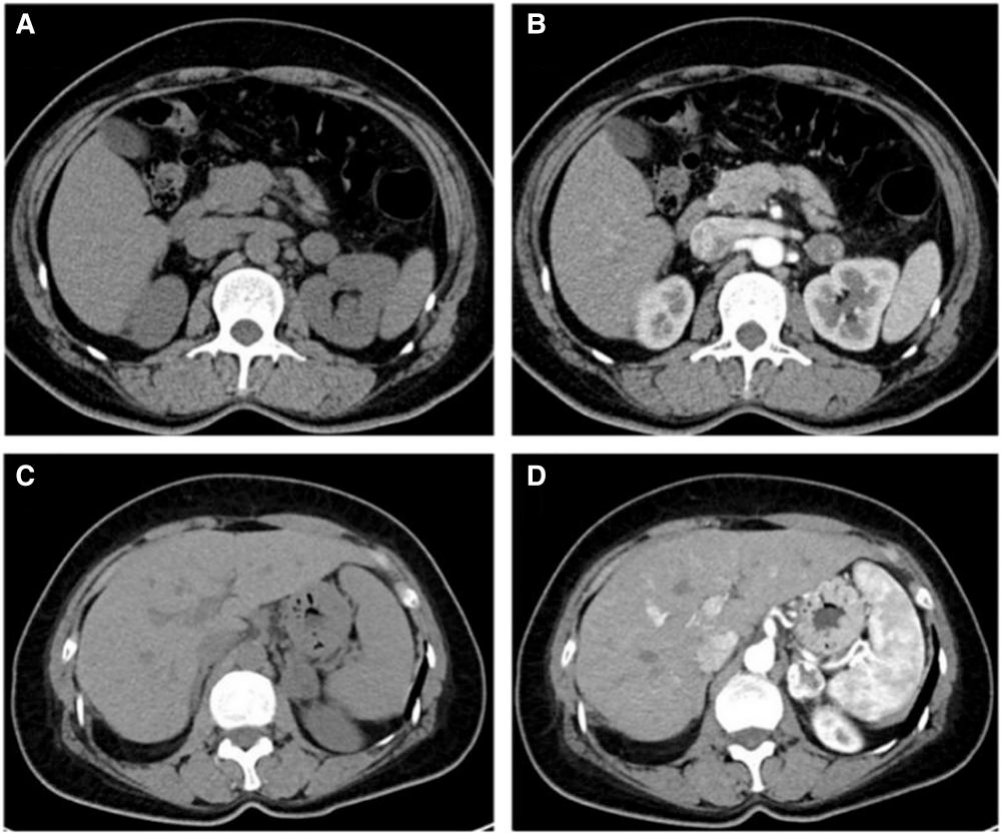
Contrast-enhanced computed tomography demonstrating different enhancement patterns of different size tumors. (A, B) shows mild enhancement of a 1.8 cm tumor and (C, D) shows marked enhancement of a 2.3 cm tumor in the arterial phase.
